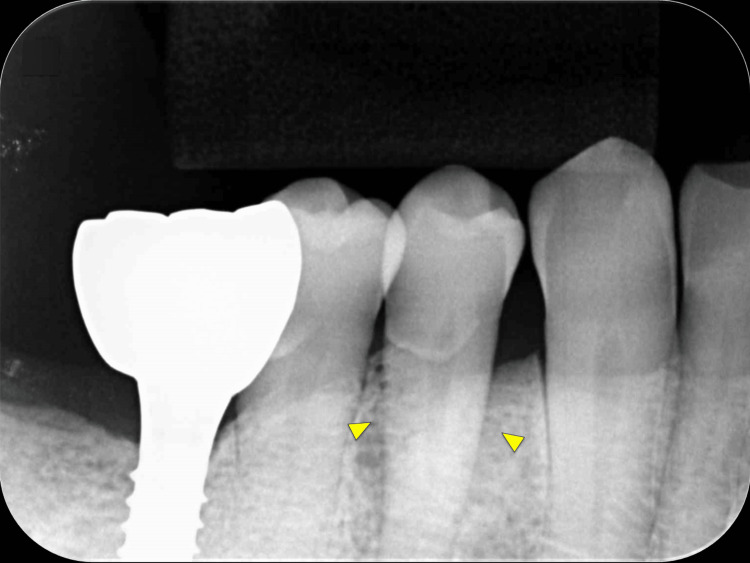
Postoperative intraoral radiographic image. Comparing only the intraoral radiographic images preoperatively and postoperatively, little change was seen in the radiolucency of the alveolar bone around tooth #44 (arrowheads).
